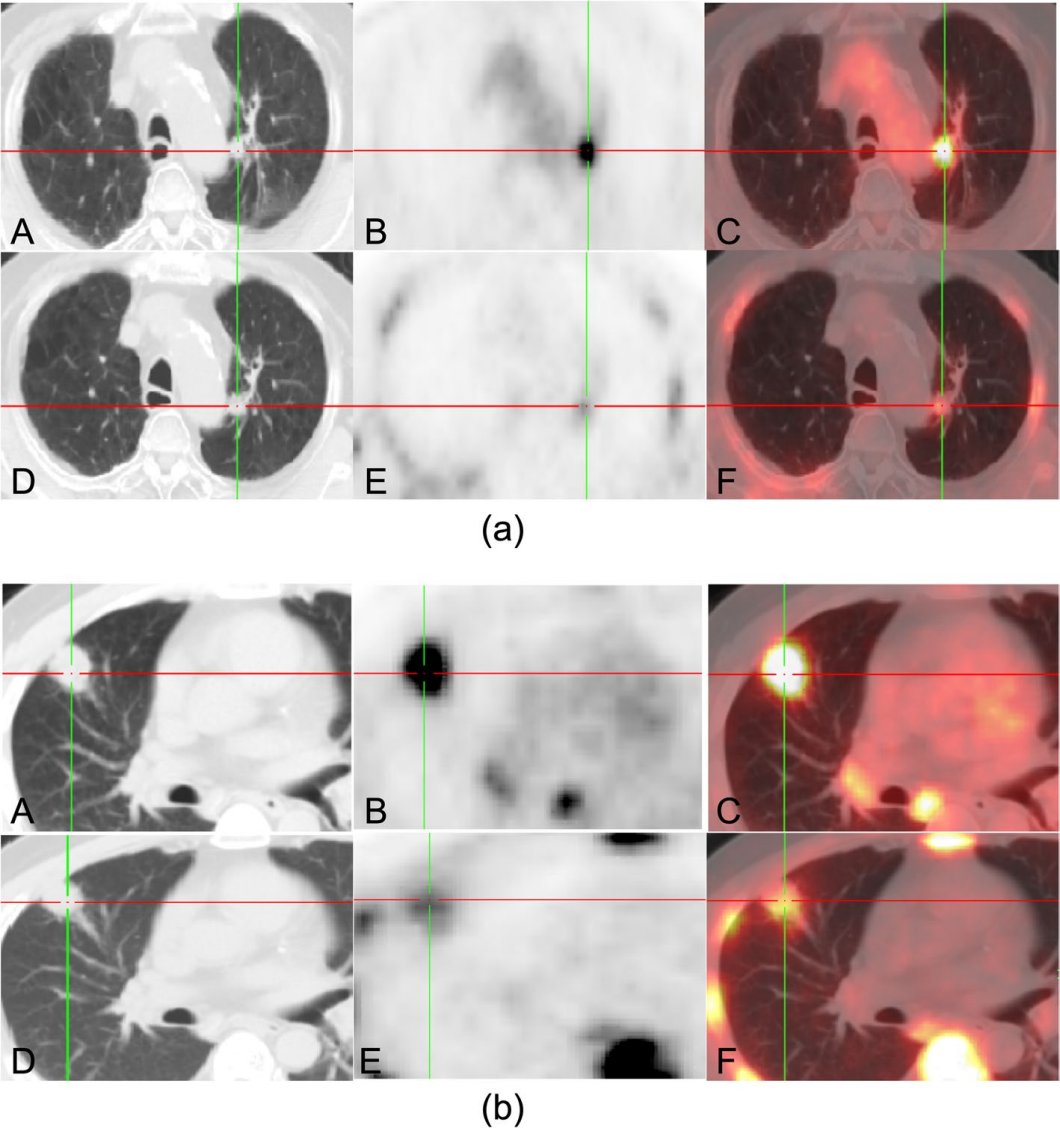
Representative dual-tracer PET/CT images of two cases. a Patient 1, male, 87 years old, malignant PN (lung cancer) (A–C). [^18^F]FDG PET/CT images show a small nodule without smooth, well-marginated borders, which lied near the aortic arch in the upper lobe of the left lung. The size was about 1.1 × 1.2 cm. Besides, higher glucose uptakes were observed within the nodule (SUVmax: 6.94) (D–F). [^18^F]FLT PET/CT images indicate higher tracer uptakes inside the nodule (SUVmax: 2.12). b Patient 2, male, 59 years old, benign PN (tuberculosis) (A–C). [^18^F]FDG PET/CT images show an irregularly shaped nodule with badly defined borders, which stood close to the pleura in the upper lobe of the right lung. The size was around 2.6 × 2.4 cm. Besides, there were tracer accumulation within the nodule (SUVmax: 8.26) (D–F). [^18^F]FLT PET/CT images indicated higher tracer uptakes inside the nodule (SUVmax: 2.48)
